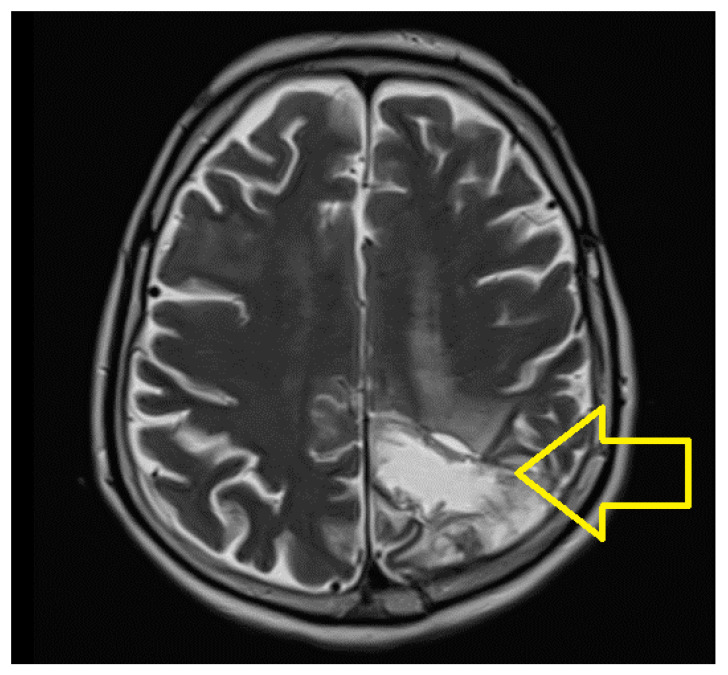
Skull MRI October 2022: left parietooccipital sequela.
